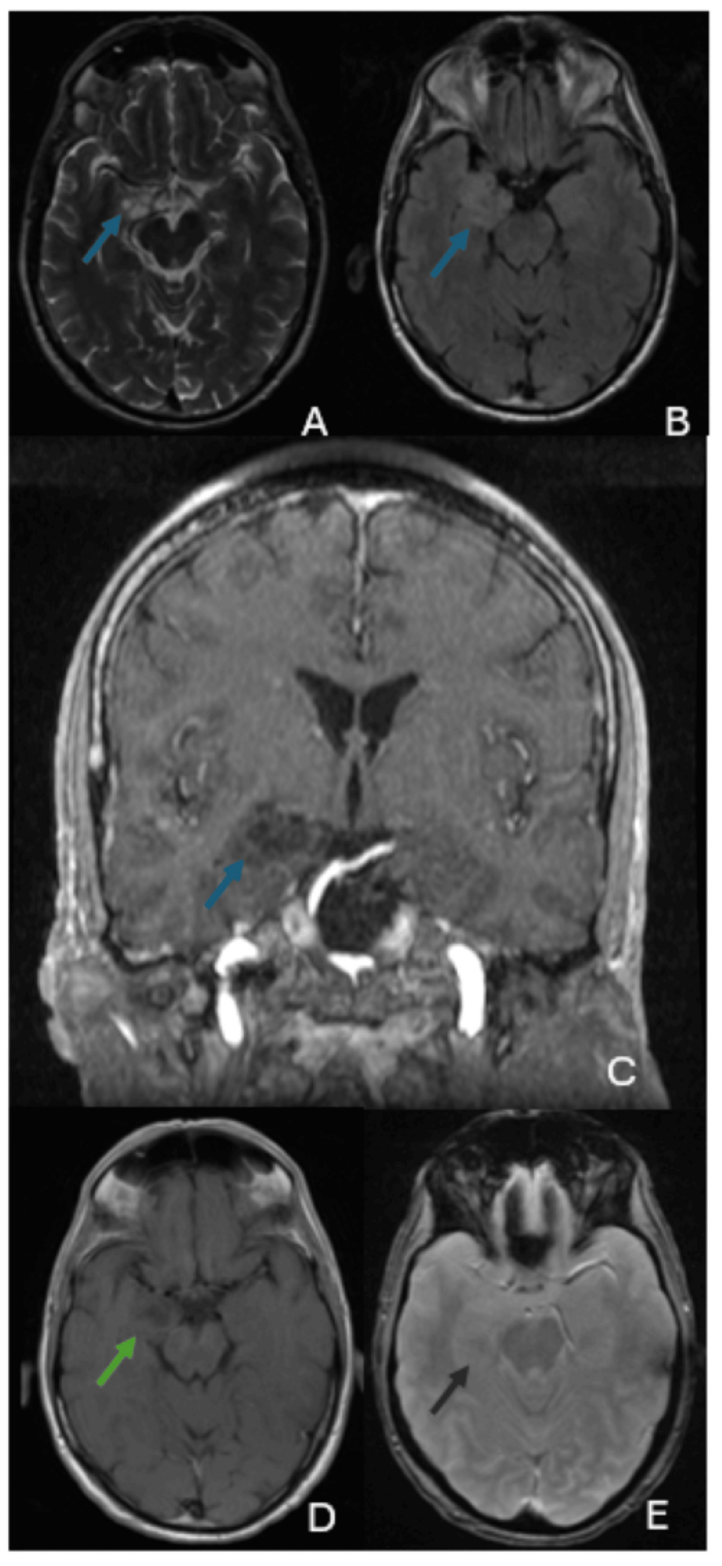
Intra-axial expansile lesion in the right mesial temporal region exhibits hyperintensity on T2-weighted and FLAIR sequences, and hypointensity on T1-weighted images. The images show multiple small cystic components resembling a "soap-bubble" appearance, with no contrast enhancement, vasogenic edema, or signs of hemorrhage or ferromagnetic substance deposition. (A) Axial T2-weighted image; (B) axial FLAIR image; (C) coronal T1-weighted image; (D) axial post-contrast T1-weighted image; (E) axial susceptibility-weighted image.
